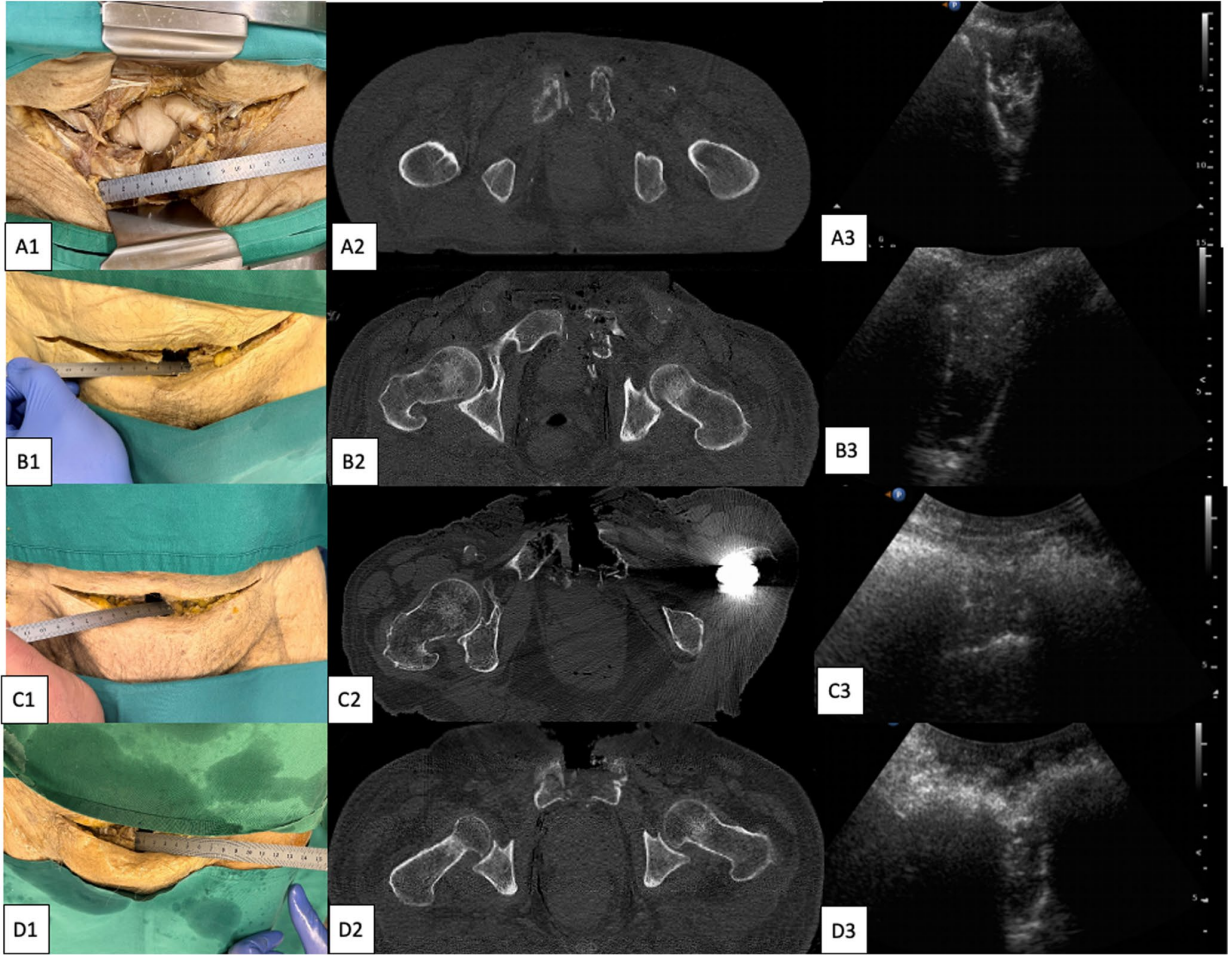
Each cadaver (A-D) with one exemplary diastasis with clinical measure, corresponding CT-scan and ultrasound image
